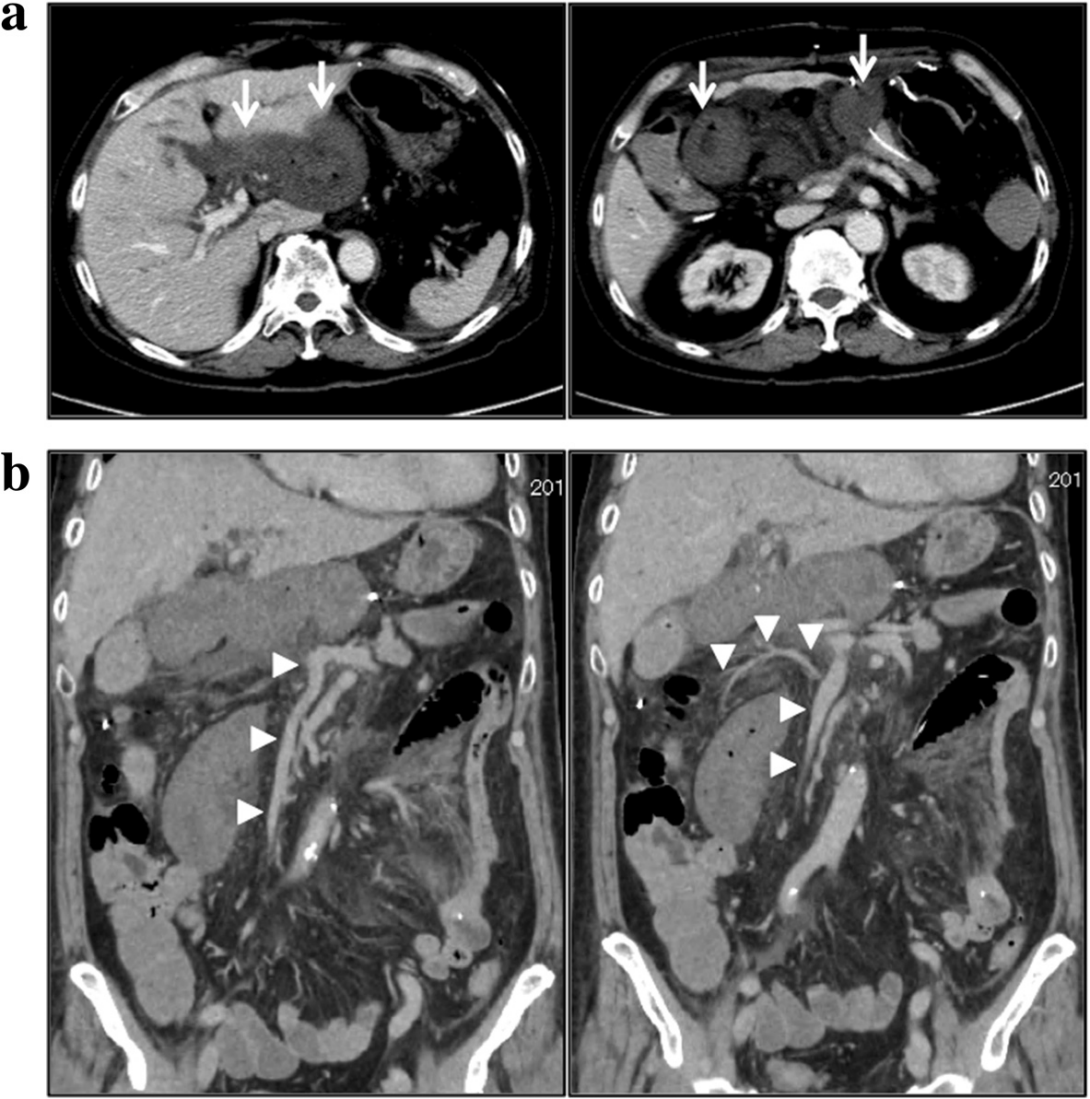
The findings of enhanced CT on POD 2: a Axial images showed acute ischemia of the jejunal loop with a thickened wall (*white arrows*). Remnant pancreas were normal. b Coronal images showed that there was no obvious thrombosis of the SMV and its branches (*white arrowheads*)
